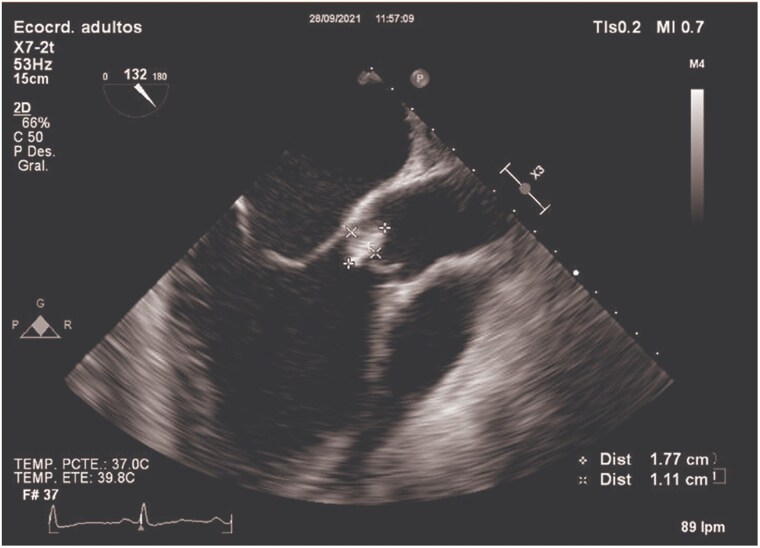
Transoesophageal echocardiography showing a 1.77 × 1.11 cm vegetation on the non-coronary cusp of the aortic valve.
